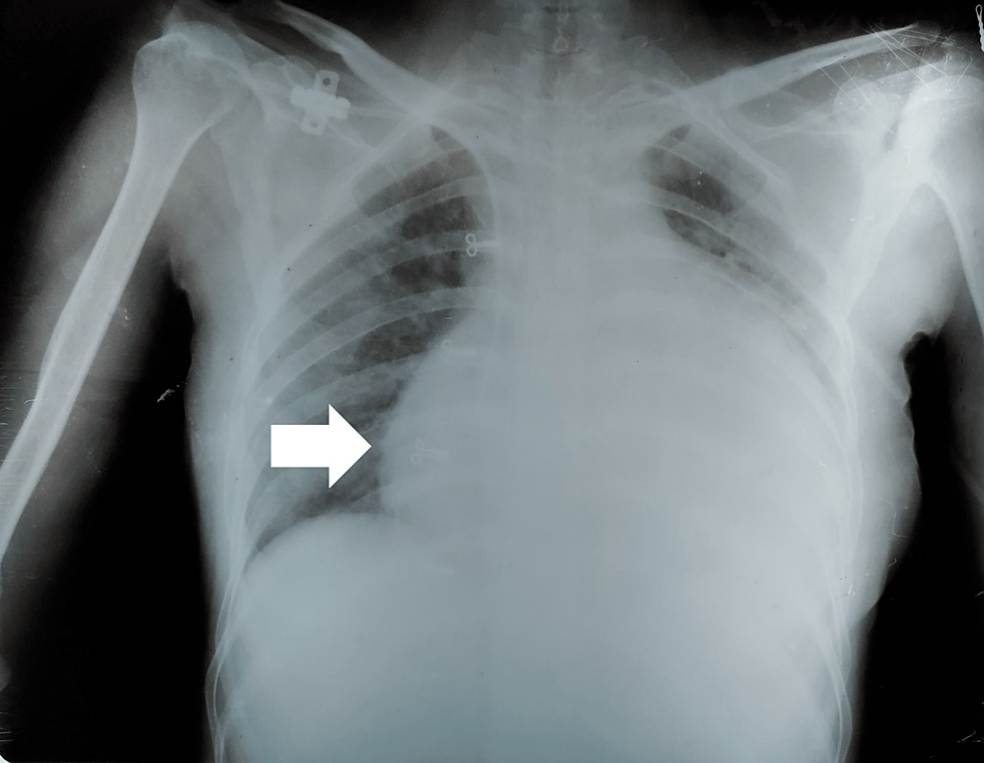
Plain chest X-ray of the patient showing enlarged cardiac silhouette (arrow)
